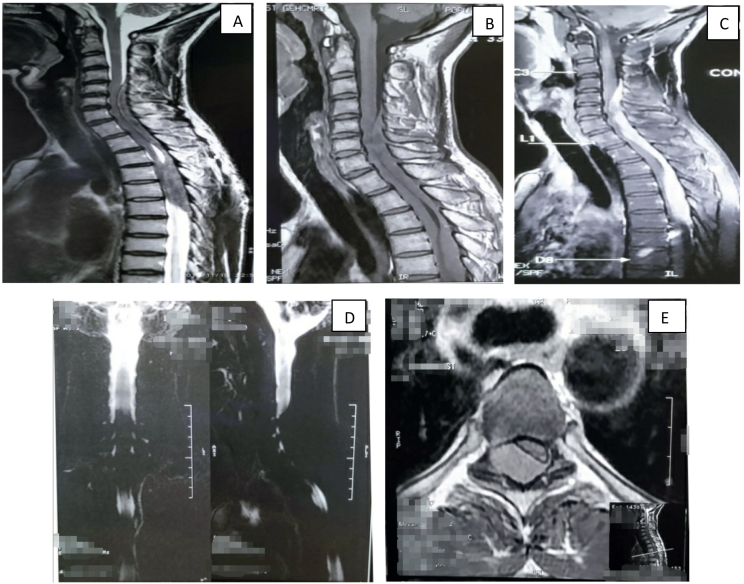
(A-E): MRI of the whole spine revealed a large elongated epidural mass in the posterior aspect of the spinal canal extending from C4-D6 levels resulting in flattening of the spinal cord, anterior displacement of line, and cord compression (Fig. 1). Enhanced T1 weighted scanning showed a homogenously distinct lesion. The lesion is isointense to the spinal cord on T1 weighted images. It was iso-to-minimally hyperintense on T2 weighted images.
